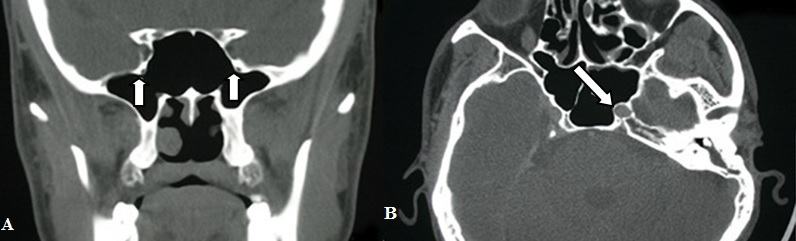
(A) bilateral foramen rotundum protrusion in coronal PNS-CT; (B) left ICA protrusion in axial PNS-CT
